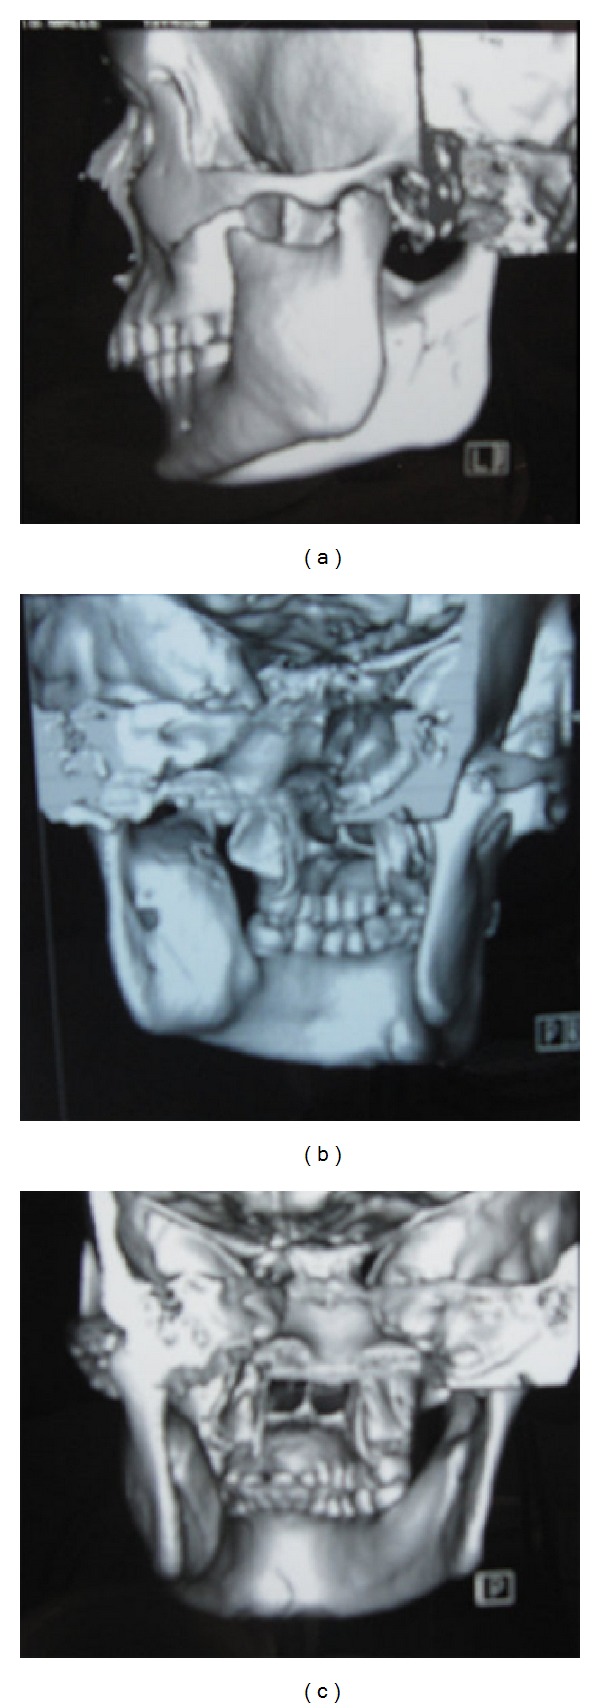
3D CT scan of the lesion showing bilaterally expansile lesion with posterior part of body and ramus of the left side of mandible.
